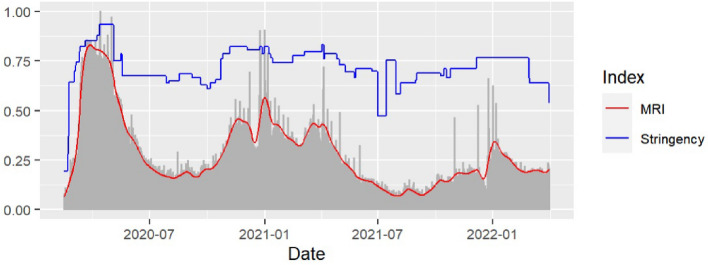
Time series of the MRI and Stringency Index for Italy from February 2020 to April 2022
